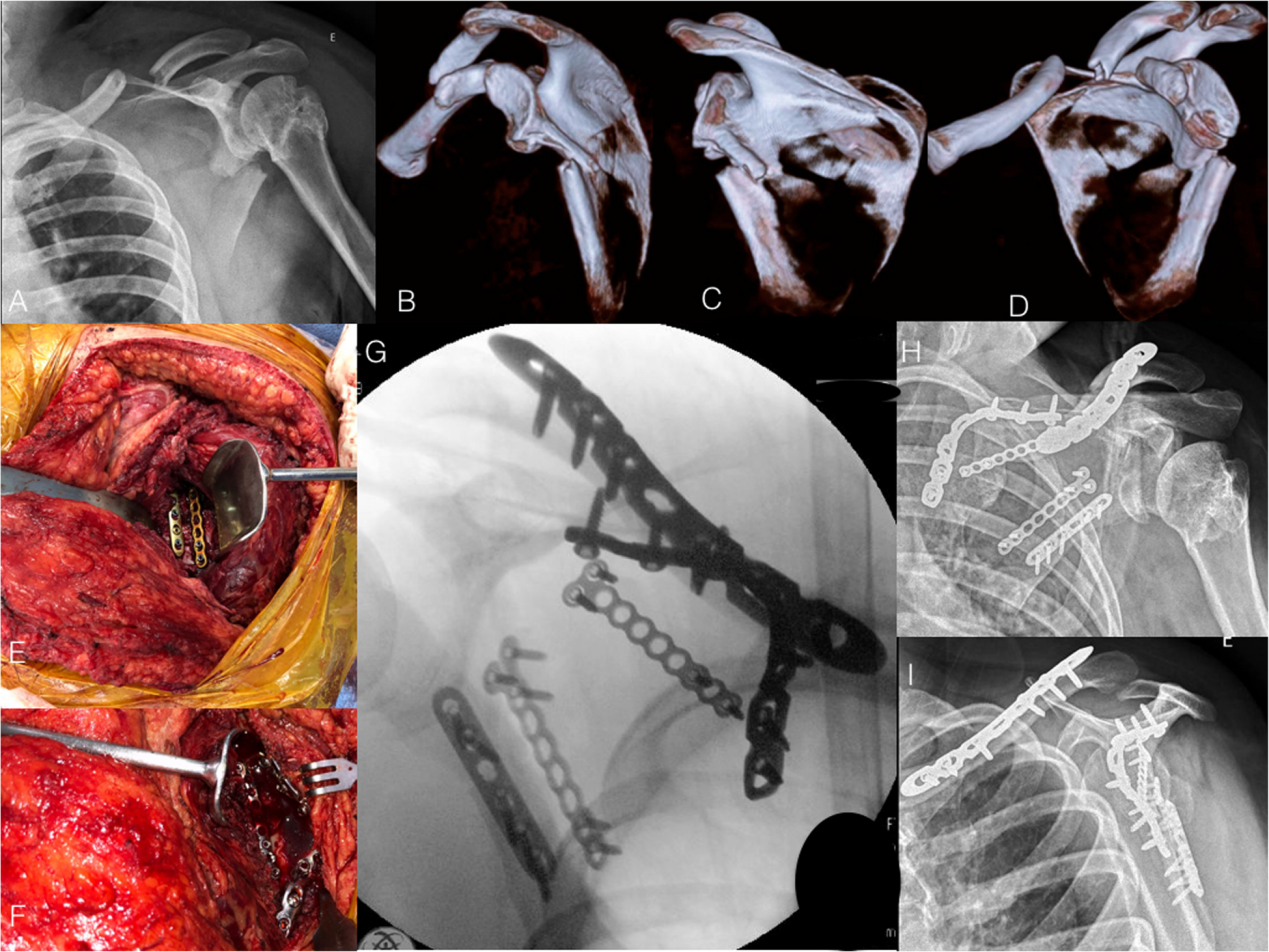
a: Radiograph of the right shoulder in anteroposterior view of a 24-year-old male patient who suffered a car accident and presented a severely displaced midshaft clavicle fracture in combination with an infraglenoid fracture of the scapula body. Observe that the patient presented a sequelae of previous proximal humeral and glenoid fractures, with no residual shoulder instability. b, c, and d: 3-D CT reconstruction showing the medialization of the glenoid and the angulation of the scapular body. e and f: Perioperative photographs depicting the modified Judet approach. Observe the fixation of the lateral pillar of the scapula with two plates at the interval between the infraspinatus and teres minor muscles (e). The medial pillar of the scapula was reduced and fixed with a twisted reconstruction locking plate. Observe the minimal detachment of the infraspinatus muscle (f). g: Perioperative fluoroscopy image showing scapula and clavicle fractures reduction and fixation. h and i: Radiographs in anteroposterior and lateral views showing fracture healing after three months
